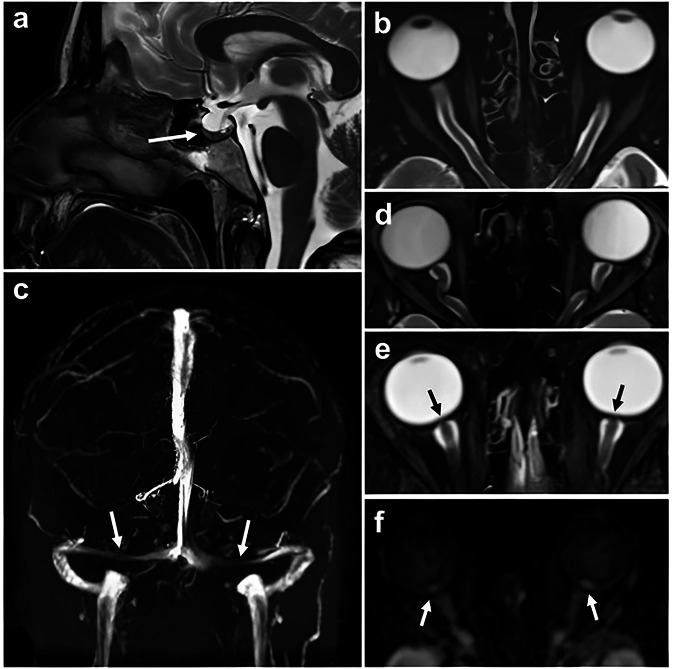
MRI features of IIH. a Mid-sagittal T2-weighted MRI shows a partially ES with downward compression of pituitary tissue (white arrow). b The axial T2-weighted image reveals a distension of the perioptic subarachnoid space surrounding both optic nerves c Time of flight MR venography shows bilateral stenoses of the transverse sinuses (white arrows). d Axial T2-weighted MRI demonstrates tortuosity of both optic nerves. e Axial T2-weighted MRI depicting bilateral PGF (black arrows). f Diffusion weighted imaging (DWI) shows bilateral hyperintensity of the optic nerve head (more prominent on the left side, white arrows)
